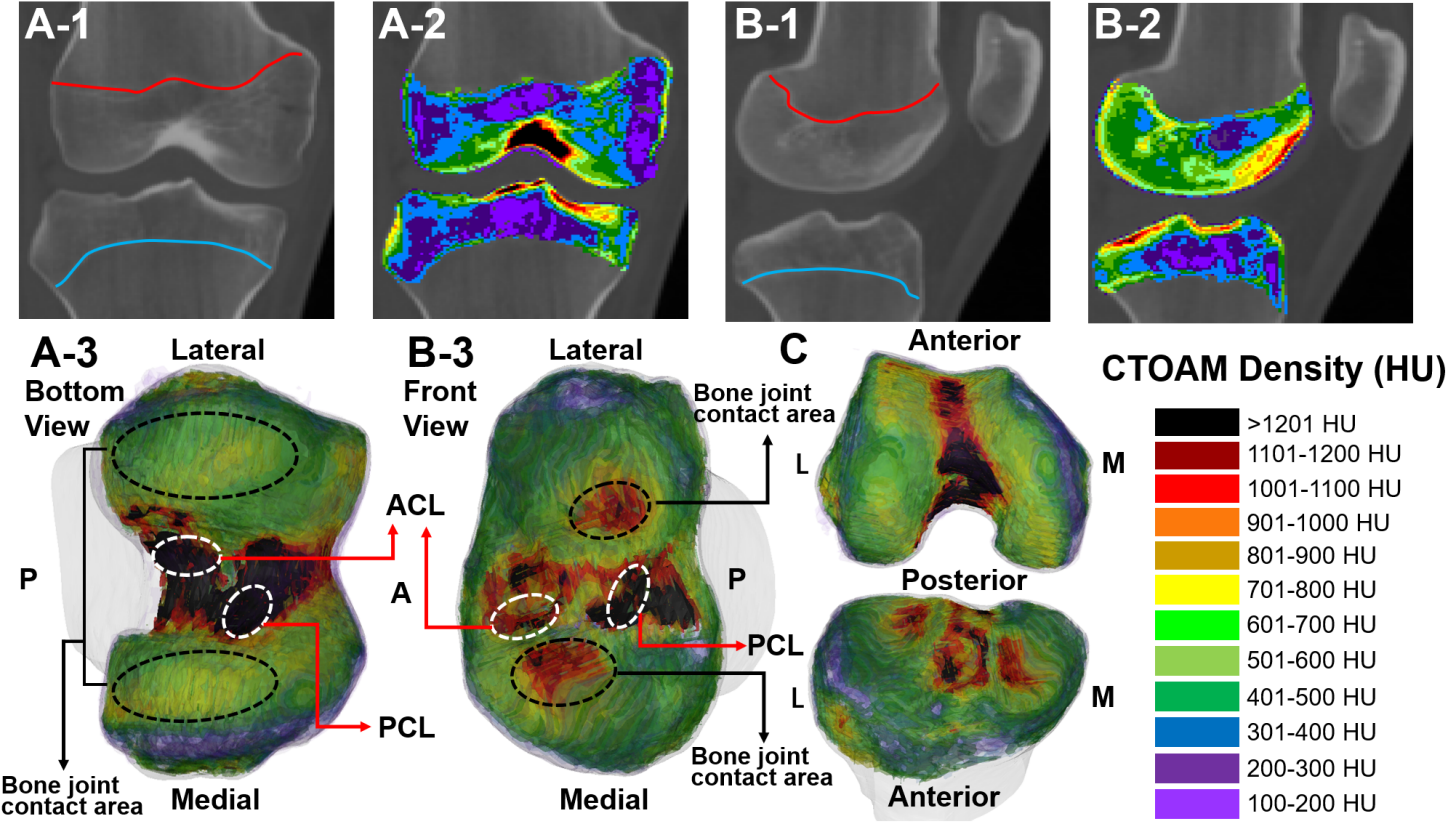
Range and post-processing reconstructed model of subchondral bone image segmentation (CT value, Hounsfield Unit, HU) of the knee joint in two subjects. (A-1, B-1) CT segmentation range of the knee the epiphyseal line; (A-2, B-2) CTOAM segmentation images of the knee epiphyseal line; (A-3, B-3) CTOAM model of the reconstructed bone tissue of the knee joint; (C) Reconstructed knee joint model after opening (front view); CTOAM image density interval. These pictures were from the judo athletes. ACL is anterior cruciate ligament; PCL is posterior cruciate ligament; M is medial; L is lateral.
